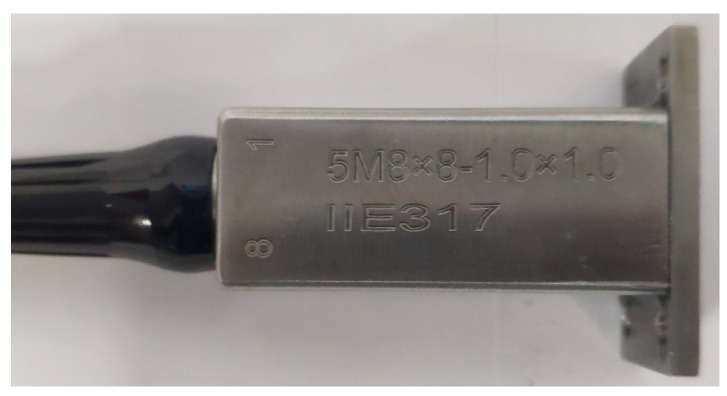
Matrix phased array Doppler 5M8 × 8BP1.0.
